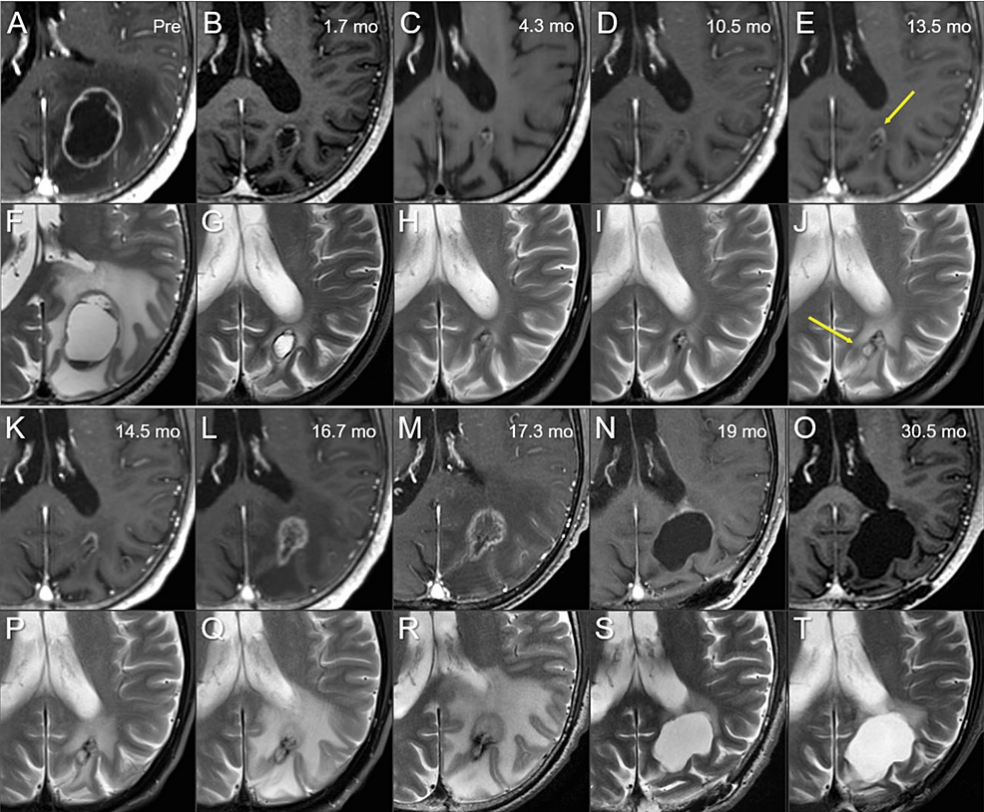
Serial magnetic resonance images for brain metastasis in the left parietal lobe. The images show (A-E, K-O) axial CE-T1-WI; (F-J, P-T) axial T2-WI; (A, F) before fSRS (pre); (B, G) at 1.7 months (mo); (C, H) at 4.3 months; (D, I) at 10.5 months; (E, J) at 13.5 months; (K, P) at 14.5 months; (L, Q) at 16.7 months; (M, R) at 17.3 months; (N, S) at 19 months; (O, T) at 30.5 months. These serial image datasets were co-registered and fused on MIM Maestro^TM^ (Cleveland, OH: MIM Software). The volumes of the visible mass on CE-T1-WI and/or T2-WI at 4.3 and 17.3 months are 0.94 and 3.19 cm^3^, respectively. At 13.5 months, increased enhancement (arrow in E) and a cystic change (arrow in J) are noted at the ventral and dorsal part of the remnant lesion, respectively. CE: contrast-enhanced; T1-WI: T1-weighted image; T2-WI: T2-weighted image
